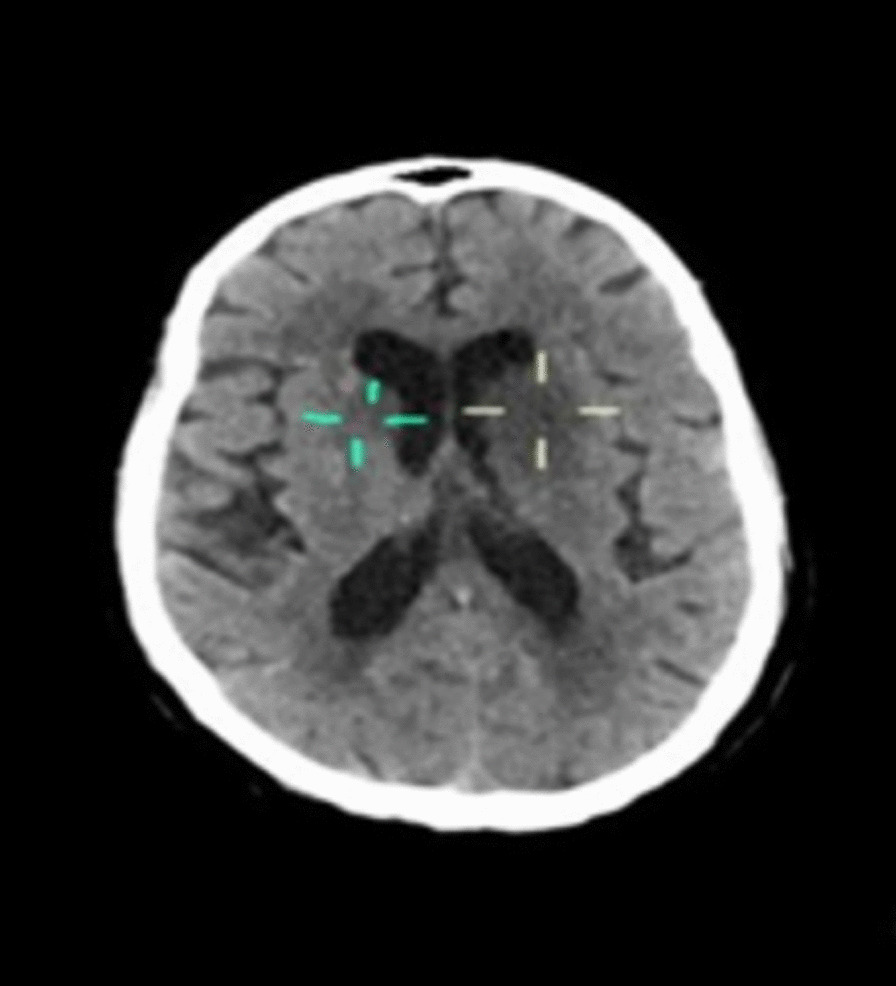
Non-contrast computed tomography (NCCT) of the brain showing accentuated hypodensities in left basal ganglia (involving caudate nucleus, lentiform nucleus, and anterior limb of internal capsule) and a smaller hypodensity in the right caudate nucleus
